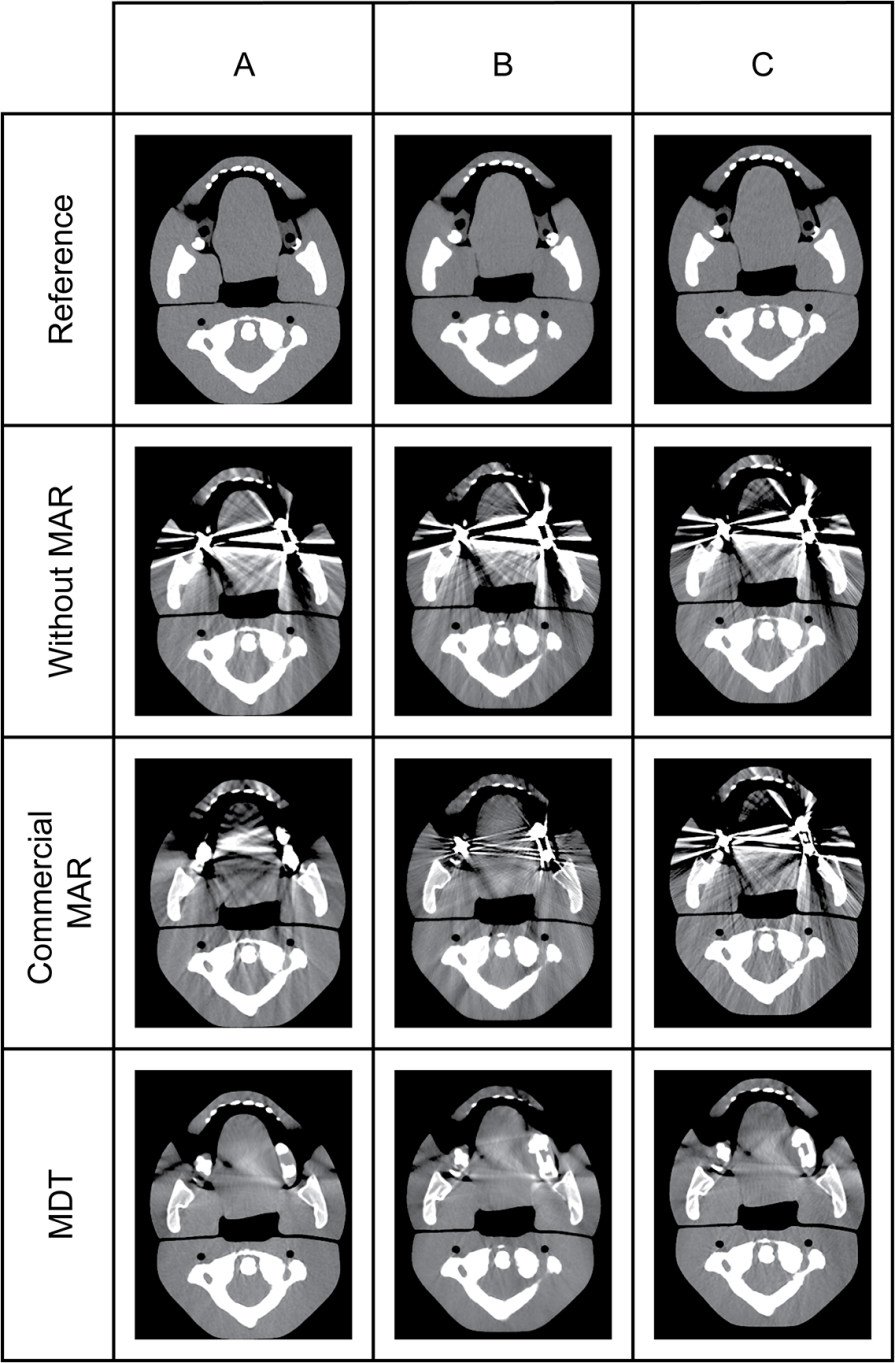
CT reconstructions made with and without metal implants and with different MAR techniques. For S3, the reconstruction using MAR2 is displayed. The window and level were set at 400 and 40 respectively. S1: Philips Brilliance ICT 256, S2: GE Discovery CT 750 HD, S3: Siemens Somatom Definition AS Open.
